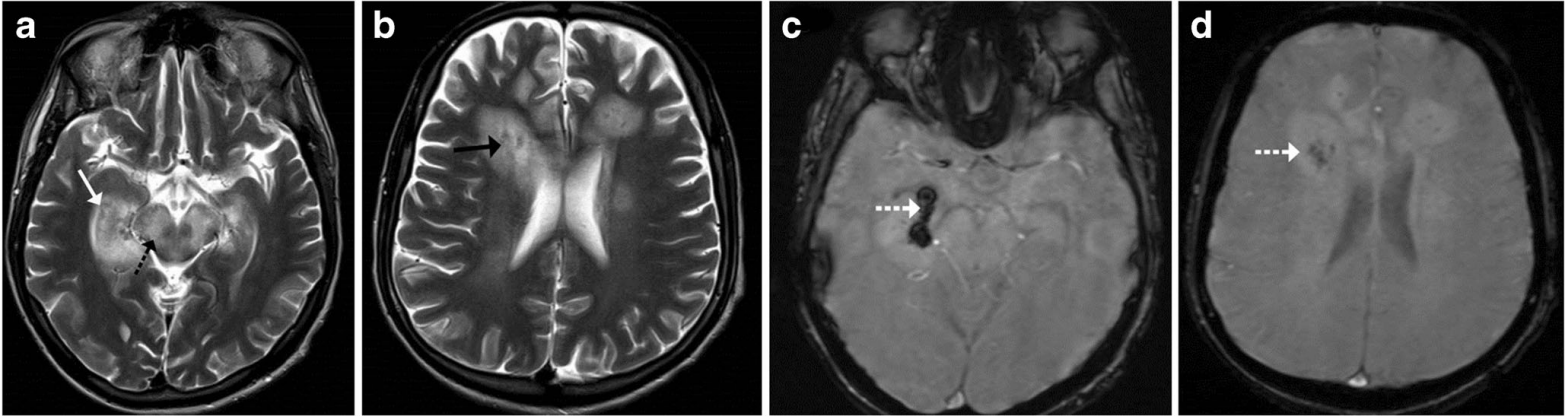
A 16-year-old patient with acute promyelocytic leukaemia on chemotherapy with angioinvasive aspergillosis: a–b Axial T2- weighted MRI shows increased signal intensity in the temporal lobes (*white arrow*) with involvement of the cerebral peduncles. Similar signal intensity is noted in the frontal white matter bilaterally. c–d Significant blooming (*dotted arrows*) is noted on susceptibility-weighted imaging
